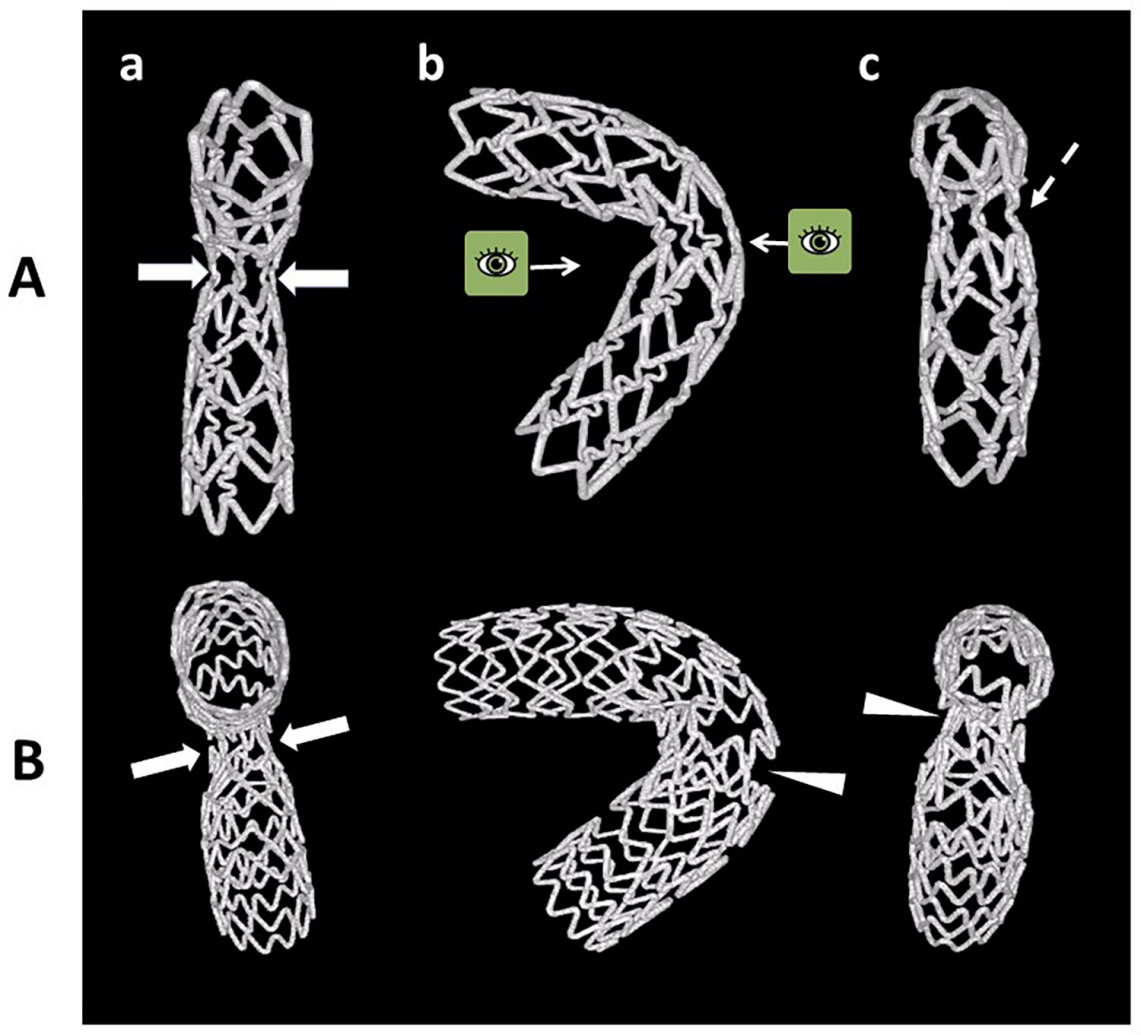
Micro-focus computed tomography images of the SB stents deployed from the MV (a: View from the inner side, b: Anterior—posterior view, c: View from the outer side). Experiments were performed using tube-slotted type stent (A) and coil type stent (B). There were restrictions of the stent expansions in both stents (A[a], B[a], arrows). The outer struts of the tube-slotted type stent were stretched (A[c], dotted arrow), whereas the coil type stent had wide opening between the coils at its outer side (B[b], [c], white triangles). Adapted from reference 7, Fig 18.
